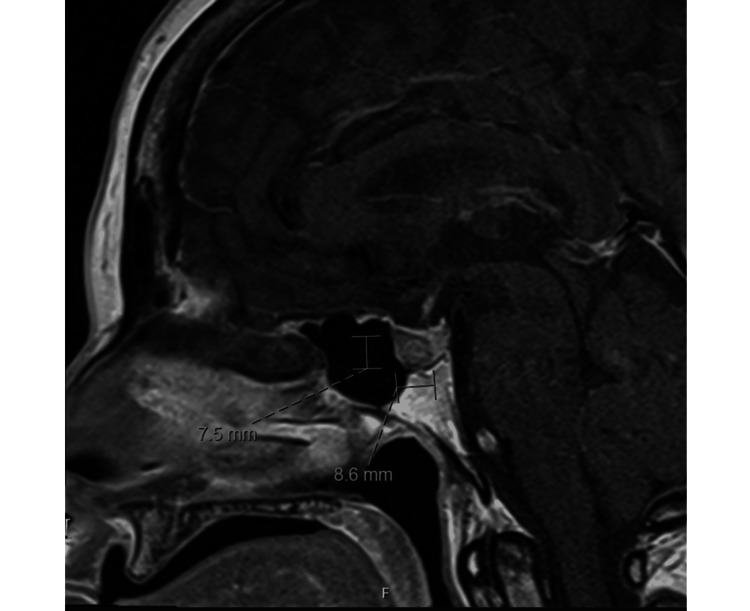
Sagittal MRI three months after initial visit and shortly before surgery, showing a 7.5 × 8.6 mm hypoenhancing lesion in the anterior sella. No suprasellar extension or optic chiasm compression.
